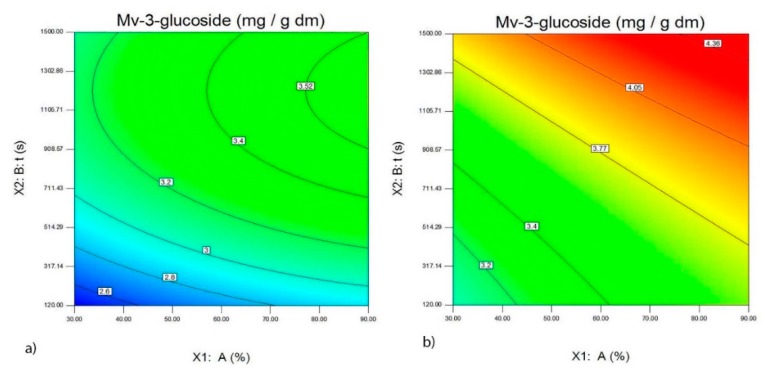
The effect of the probe with a diameter of 22 mm, amplitude A (%) and the time of ultrasound extraction t (s) on the quantity of Mv-3-glucoside (mg/g d.m.) in the extracts of the wine lees of the Merlot variety (a) and the wine lees of the Vranac variety (b).
